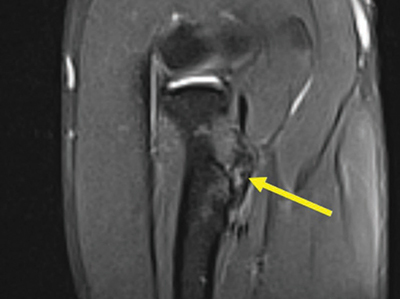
Coronal fat-saturated T2-weighted image at the level of the biceps tendon insertion with an irregular T2-hyperintense signal within, score 2.
